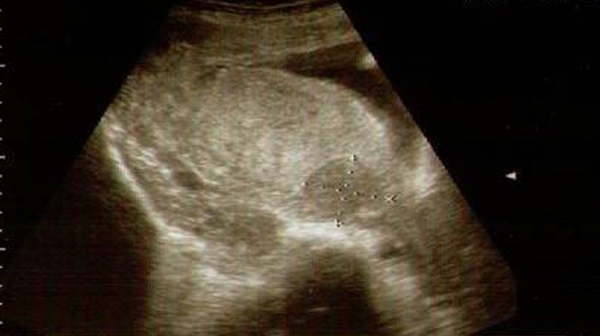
A hypoechoic, 3.6 × 4.2 cm rounded mass within the placenta on ultrasound examination at 36 weeks' gestation.
